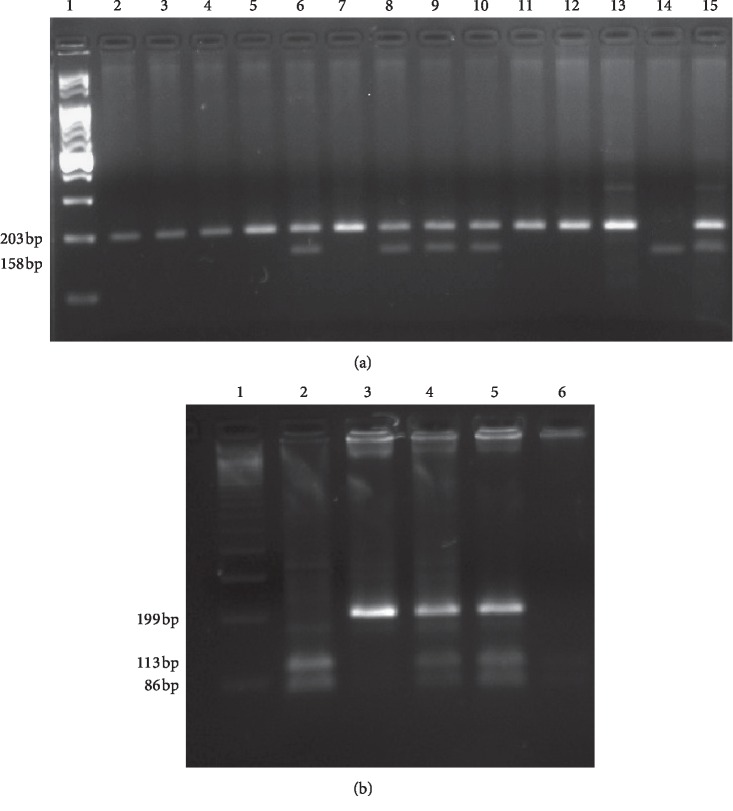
PCR-RFLP of studied polymorphisms. (a) T6746C *NOTCH3* polymorphism. Lane 1: Ladder (100 bp); Lanes 2–5, 7, and 11–13: TT genotypes; Lanes 6, 8–10, and 15: CT heterozygotes; Lane 14: CC homozygote. (b) P72R *TP53* polymorphism. Lane 1: Ladder (100 bp); Lanes 2 and 6: RR genotypes; Lane 3: PP genotype; Lanes 4 and 5: PR heterozygotes.
